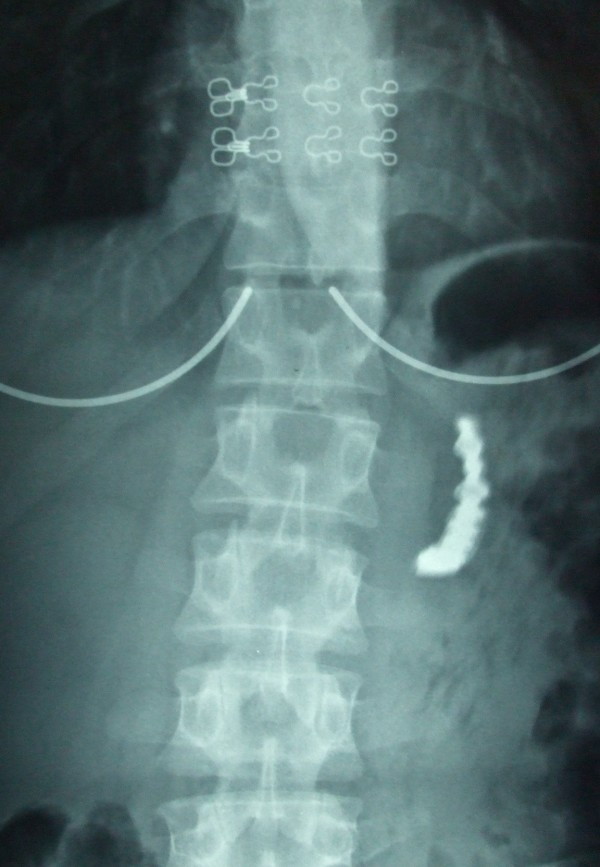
Image of partial denture at left upper quadrant of the plain abdominal radiograph on admission day.
